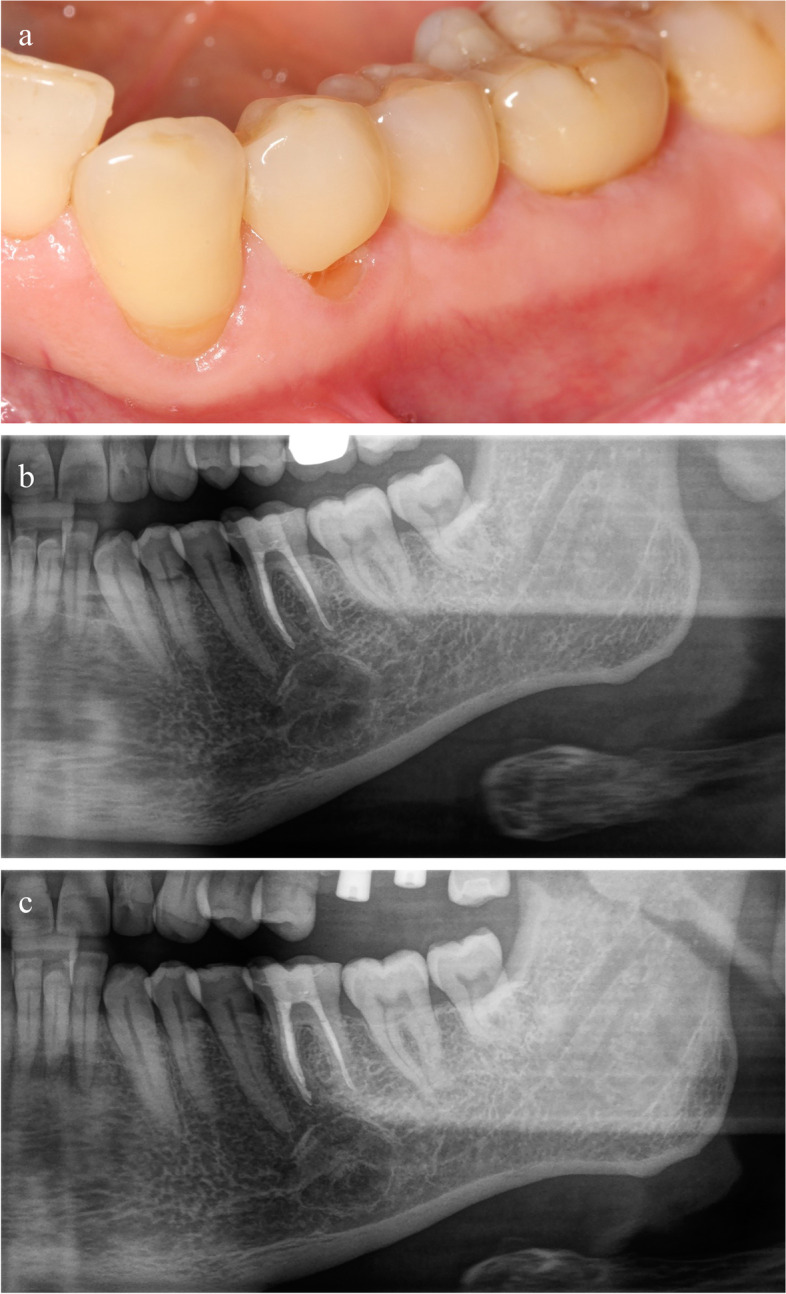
Intra-oral color photograph and partial panoramic radiographs follow-ups. a Clinical audit 7 months post-surgery. b Partial panoramic x-ray 7 month postoperative. c Partial panoramic x-ray forty-five months postoperative
